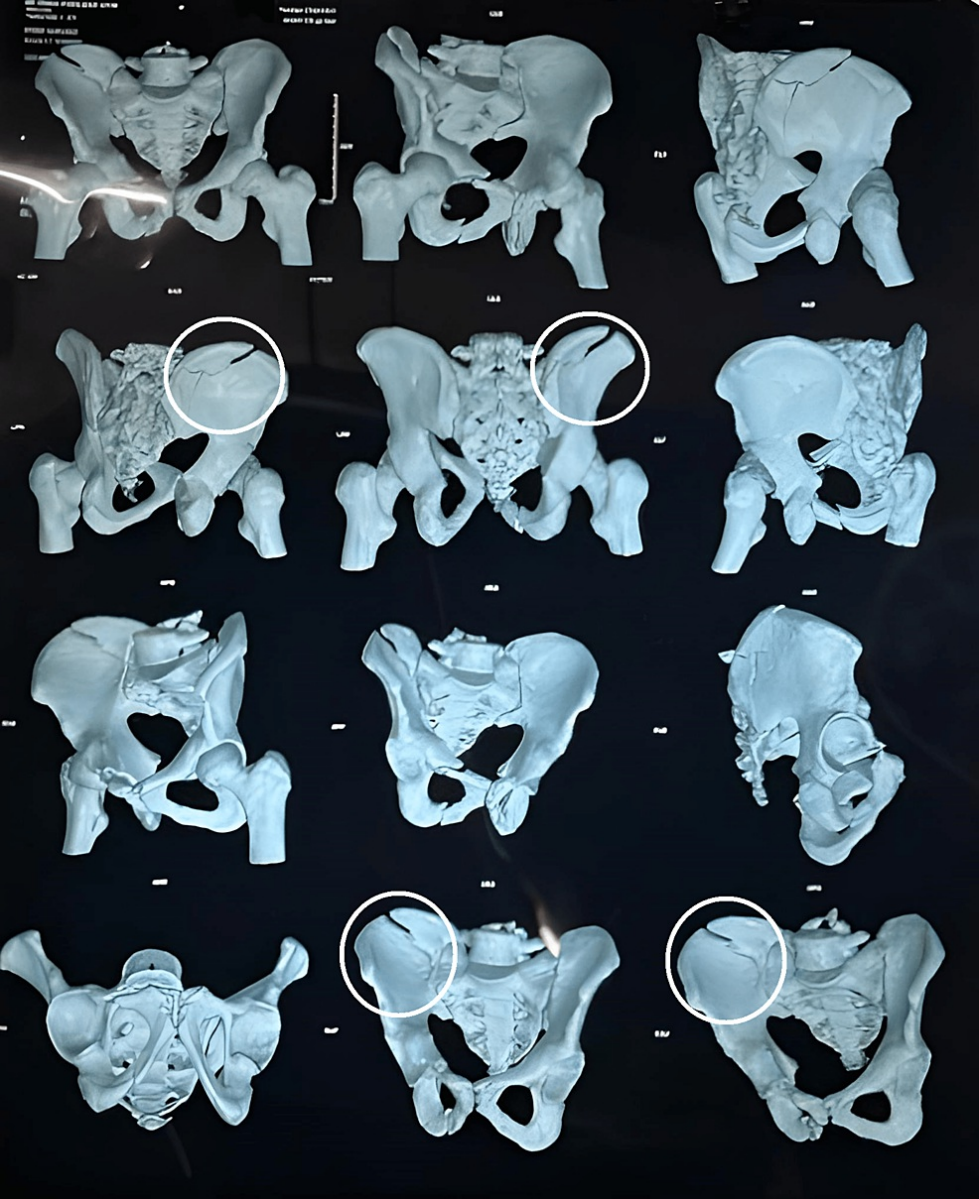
MRI of the patient's hip, with fractures part circled
